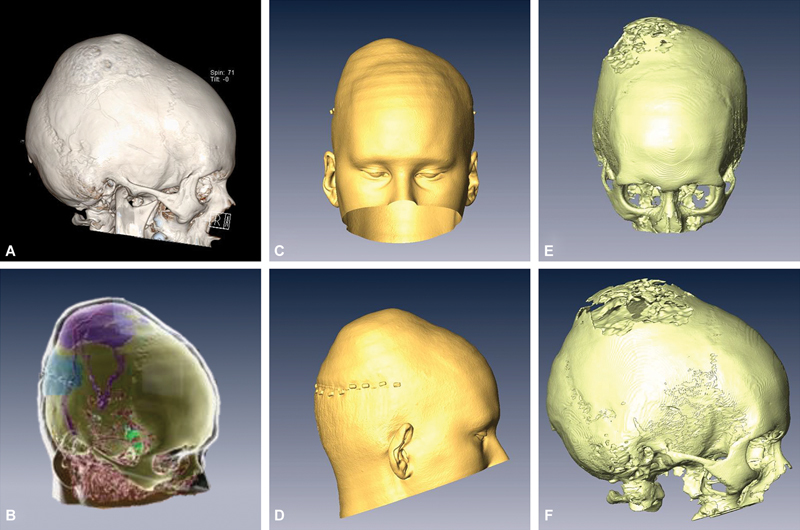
Preoperative imaging and 3D ARN model creation. CT with 3D reconstruction demonstrates fibrous dysplasia of the superior aspect of the right parietal bone (
